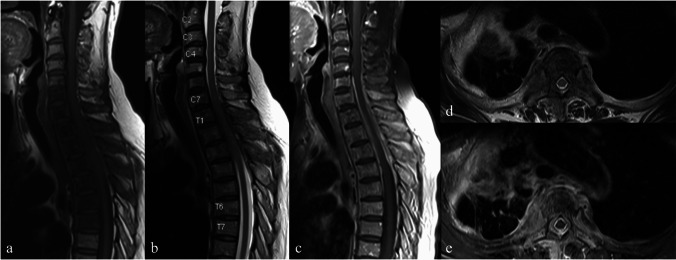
Magnetic resonance images of a 64-year-old female patient with a history of old pulmonary tuberculosis, which later developed into aspergillus spondylitis. Sagittal T1-weighted (a), sagittal T2-weighted (b), and fat-suppressed contrast-enhanced T1-weighted (c) images reveal multiple vertebral involvement from C5 to T7, with subligamentous spreading and perivertebral and epidural soft tissue compressing the cervical spinal cord. Axial T2-weighted (d) and fat-suppressed contrast-enhanced T1-weighted (e) images at the T3-T4 level, bilateral paraspinal involvement, more prominent on the right side which also involves adjacent pleura, right apical lung, and right rib are also demonstrated
